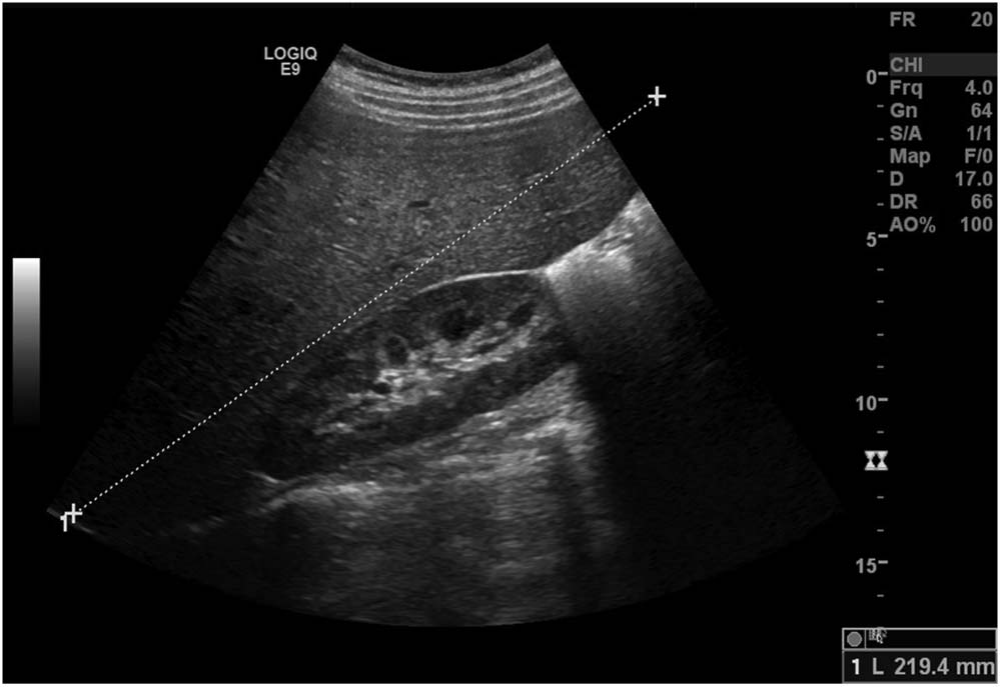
Abdominal ultrasonography revealed a liver without focal lesions, with a round border, homogeneous structure, preserved echogenicity, and increased dimensions (22 cm), causing epigastric swelling.
